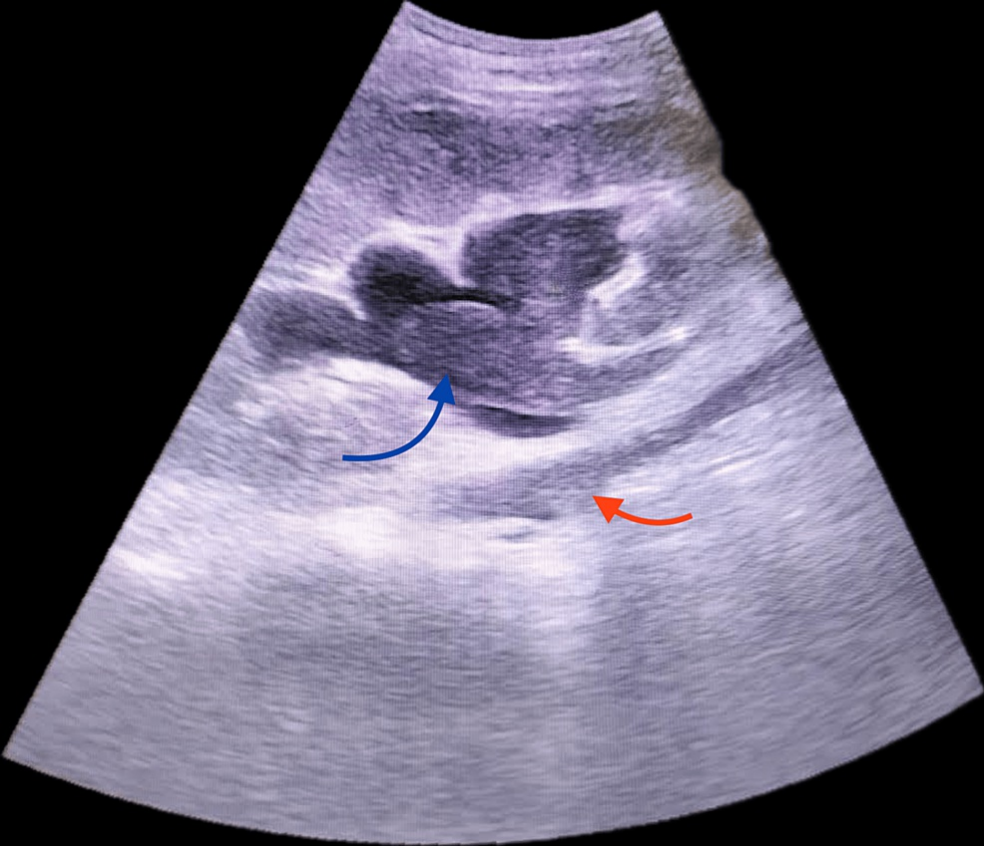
Ultrasonography of the transplant graft kidney Ultrasonography shows hydronephrosis, with an echogenic material filling the renal pelvis and continuing into the ureter (indicated by the arrow) and a collection below the ureter (indicated by the red arrow).
